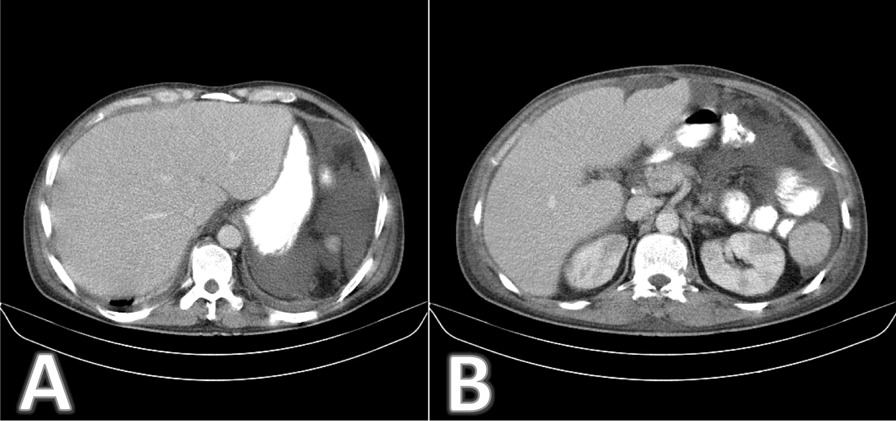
A and B: Abdominopelvic computed tomography with intravenous and oral contrast. Enlarged liver and accessory spleen, hypodense infiltrative lesions in both kidneys, multiple paraaortic lymph nodes (up to 12 mm), and severe ascites
